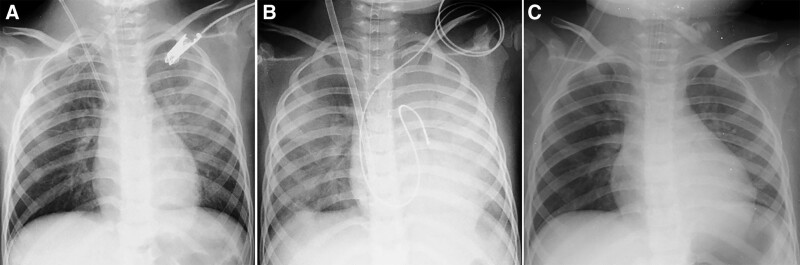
Chest radiography showed substantial bilateral pulmonary interstitial congestion on PICU admission (A) at the start of extracorporeal membrane oxygenation (VA-ECMO) (B), and marked improvement after VA-ECMO (C).
